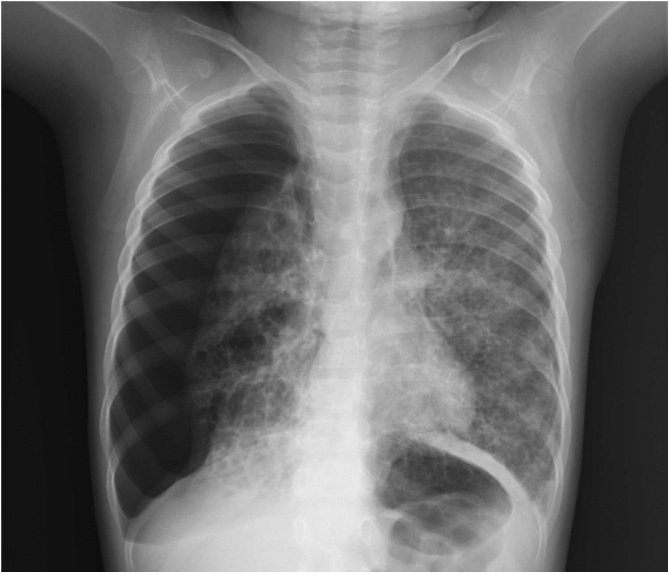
Chest X-ray after chest drainage revealed a right PTX and bilateral bullous lesions at the lung apex.
